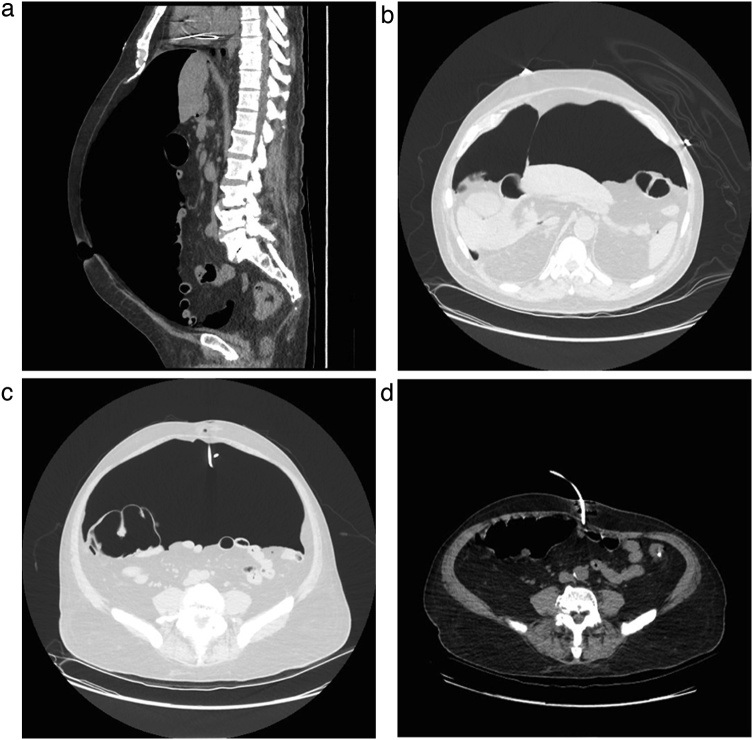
Abdominal CT-scan. a. Sagittal view showing the presence of air in the peritoneal cavity with posterior displacement of the intra-abdominal contents b. Axial view, showing the Falciform ligament sign (white arrow). c. Axial view showing percutaneous decompression with a multipurpose drainage catheter (white arrow) in the periumbilical position. d. Axial view after percutaneous decompression.
